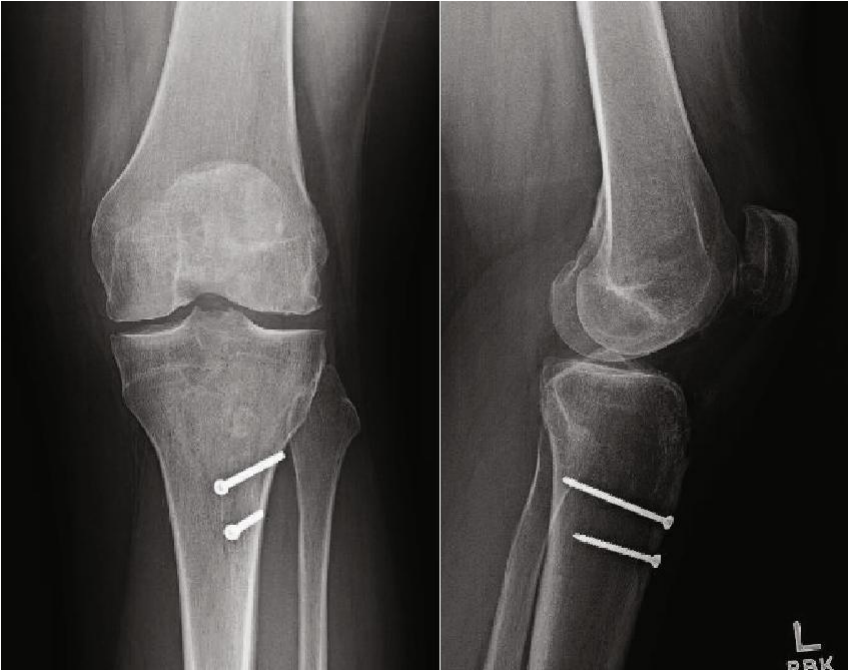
AP and lateral radiograph taken at 1-year follow-up showing healed fracture and no evidence of hardware complications.
